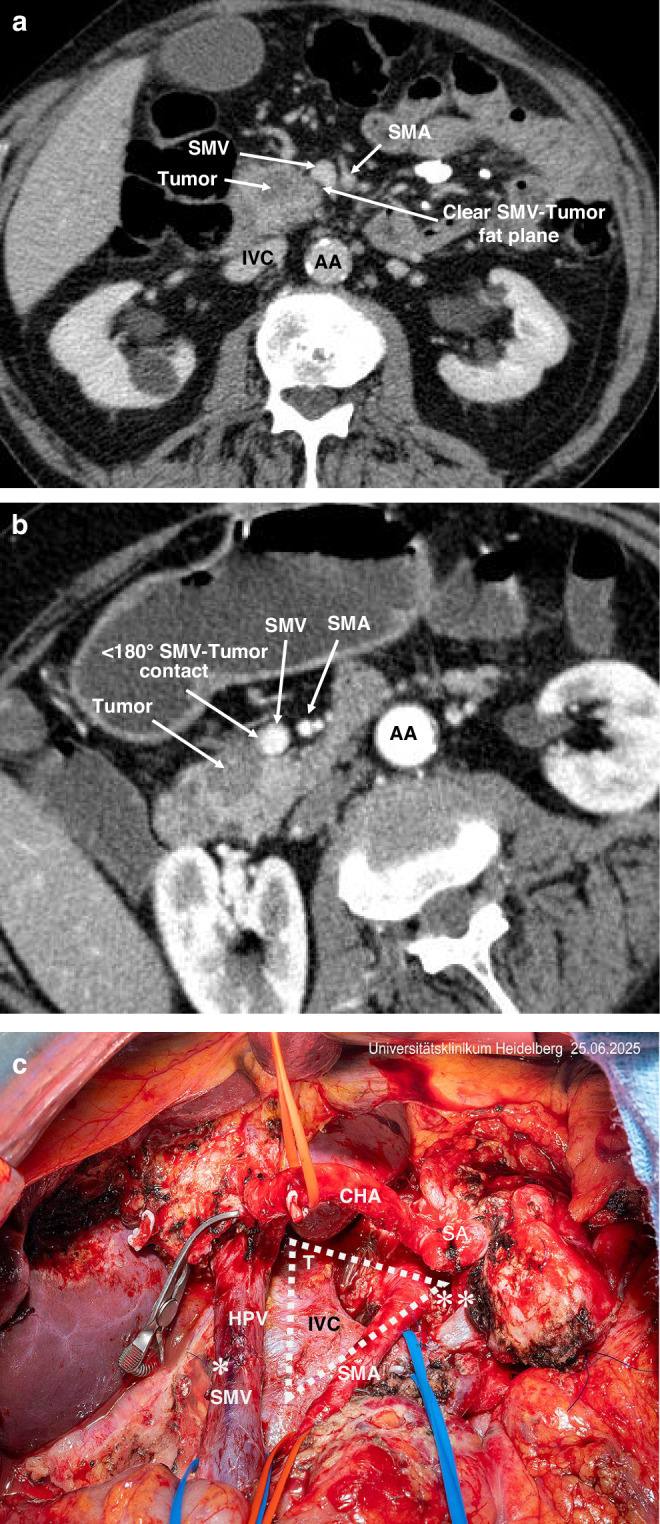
Preoperative co-axial imaging by CT demonstrating the distinction between resectbale (EmR) and borderline (EmBR) PDAC, and the key features of the TRIANGLE operation. a CT image of resectable cancer in the head of the pancreas. b CT image of borderline resectable cancer in the head of the pancreas. c Surgical site after partial pancreato-duodenectomy with venous resection, triangle dissection and splenorenal shunt for borderline rectable disease following induction chemotherapy disease. AA abdominal aorta, CHA common hepatic artery, SMA superior mesenteric artery, HPV hepatic portal vein, SMV superior mesenteric vein, SA splenic artery, IVC inferior vena cava, * hepatic portal vein resection,  splenorenal shunt, T triangle.
